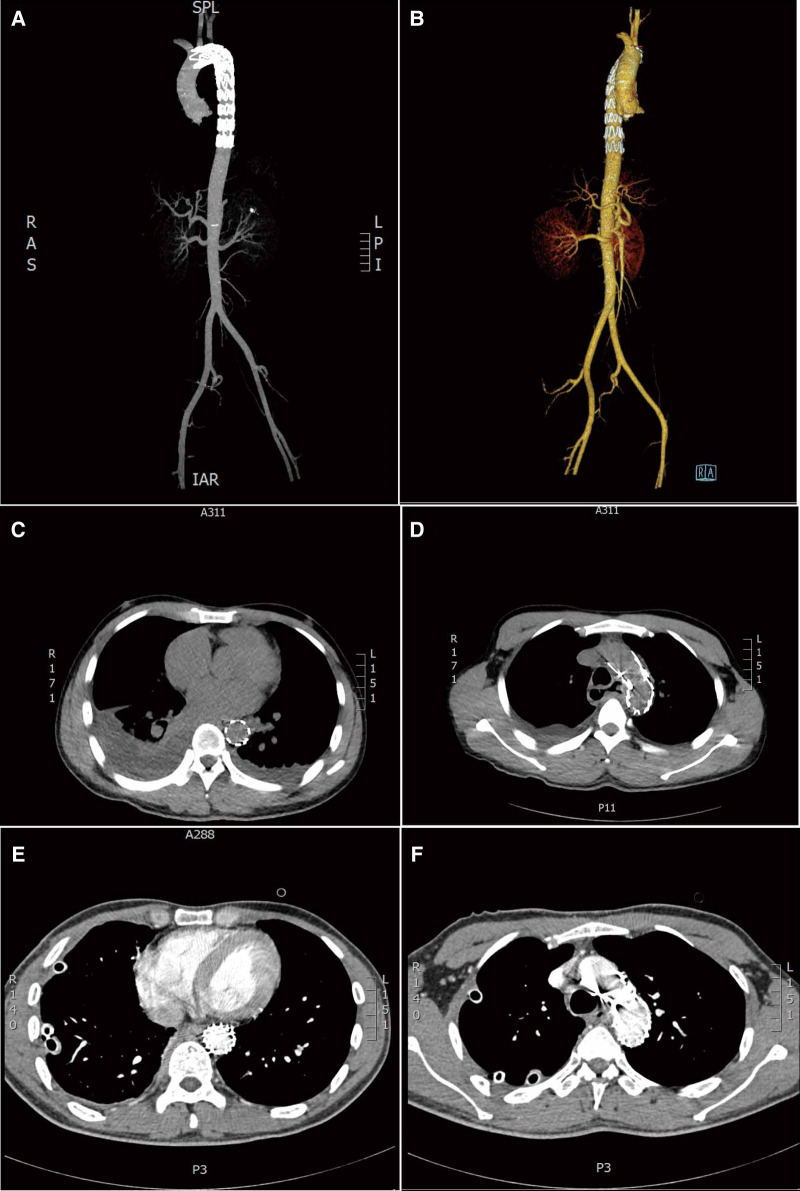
Postoperative images. (A and B) different views of three-dimensional computed tomography angiography (CTA): complete sealing of the break in the aortic wall after thoracic endovascular aortic repair (TEVAR). (C,D) Transverse view: complete sealing of the break in the aortic wall, no contrast agent extravasation was observed around the stent after TEVAR; bilateral pleural effusions were seen mainly on the right side. (E,F) Axial contrast-enhanced CT image after mediastinal debridement and drainage with cervical incision and video-assisted thoracoscopic surgery: the stent and drainage tube were observed clearly, with no fluid on both sides of the chest.
